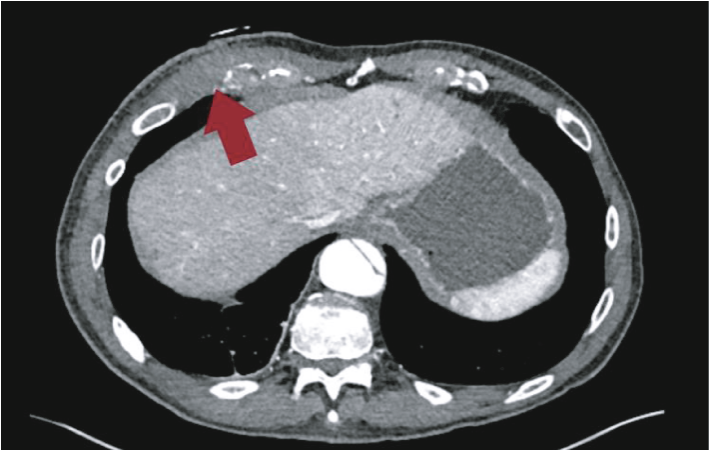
CT Scan showing fluid collection (red arrow).
